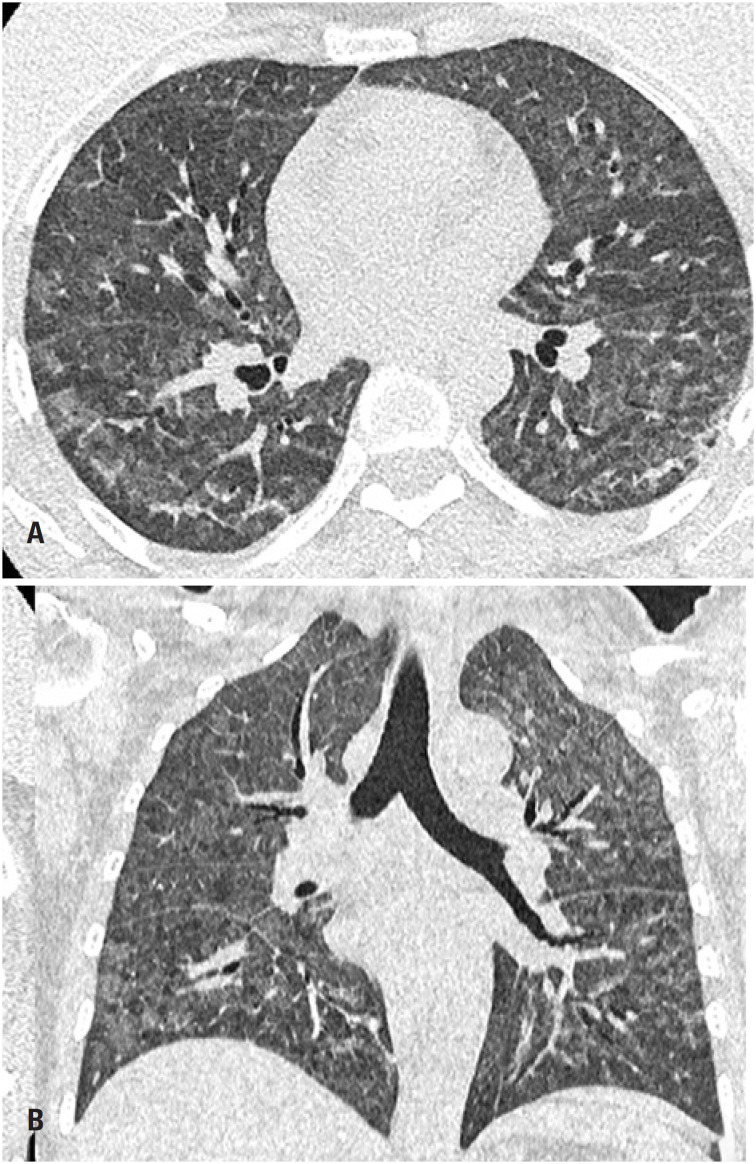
A 46-year-old woman presented with productive cough. She reported history of AIDS. Computed tomography (A) Axial section and (B) Coronal reformation image: extensive opacities affecting the pulmonary fields diffusely, with a heterogeneous ground-glass pattern associated with slight septal thickening. There was no pulmonary consolidation
